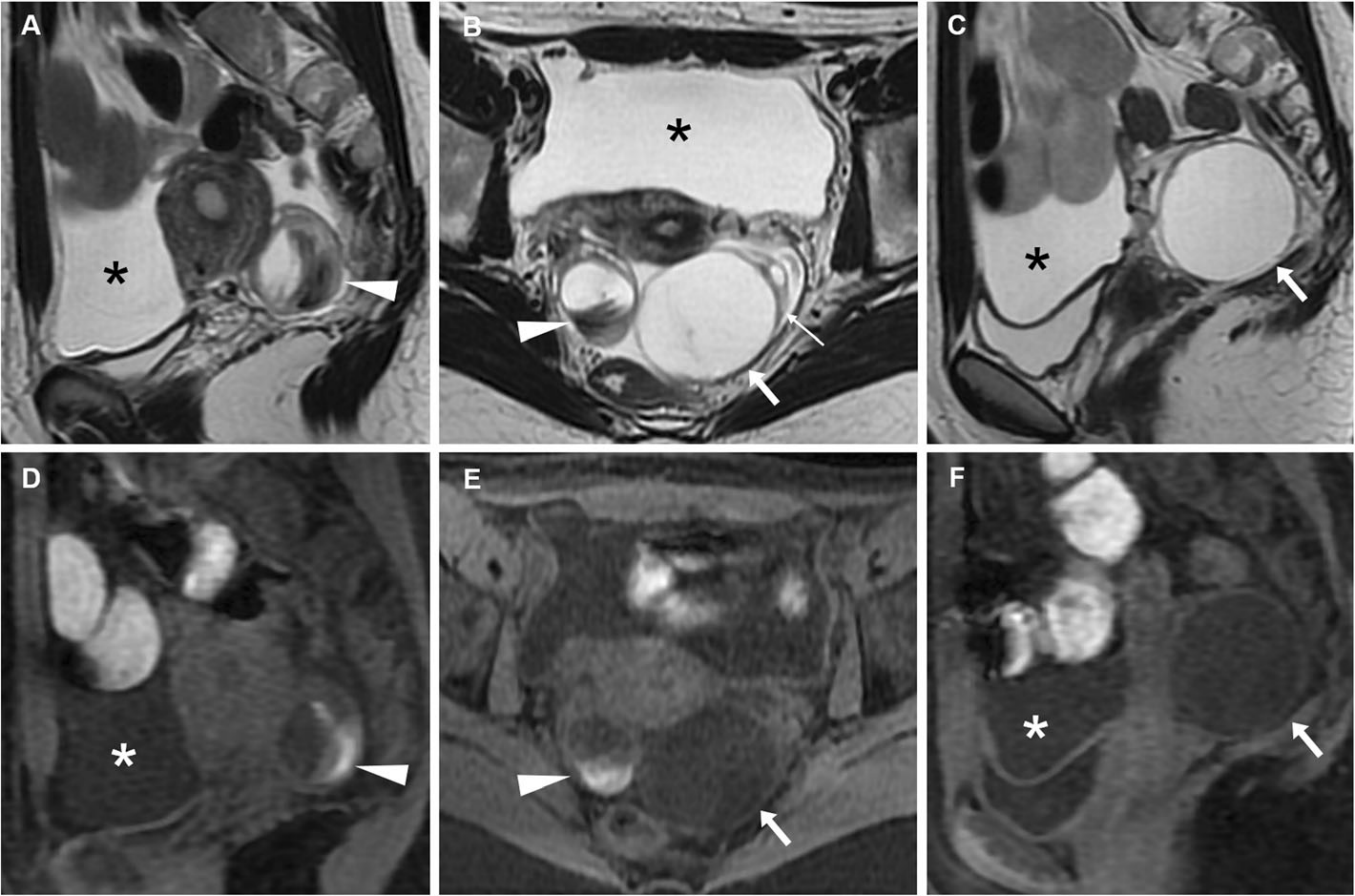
Haemorrhagic corpus luteum and functional cyst in a 15-year-old woman with pelvic pain. Multiplanar T2-weighted (a–c) and precontrast fat-suppressed T1-weighted (d–f) images showed a right adnexal cyst (arrowheads) with internal fluid-fluid level and a bloody dependent component, T2-hypointense and T1-hyperintense. Contralaterally, a well-marginated unilocular cystic lesion of the left ovary (arrows) shows thin walls and homogeneous fluid-like signal intensity. Note the compressed ovarian parenchyma along the lateral edge of the cyst (thin arrow in b) and pelvic peritoneal effusion (asterisk)
